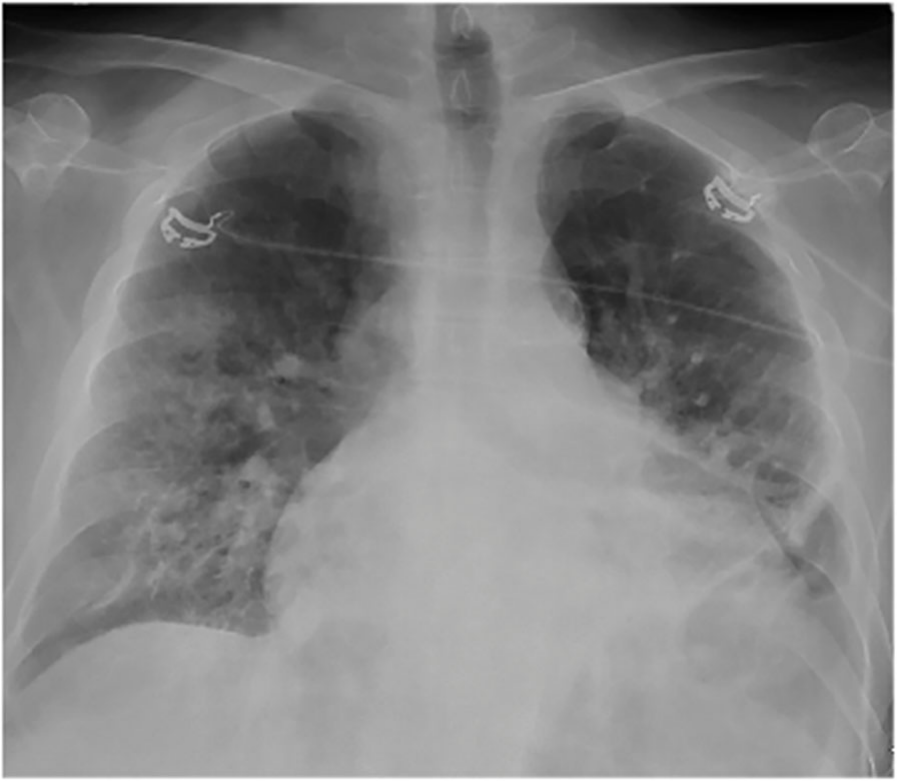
Anteroposterior chest X-ray showing new right midlung consolidation and left lower lung opacities
